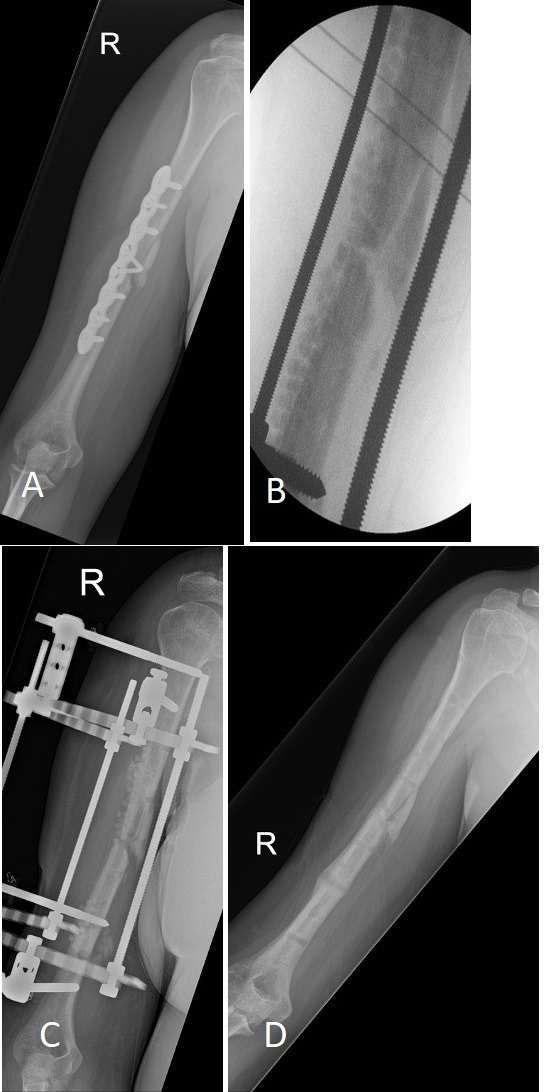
a) 39-year-old female type A host with infected nonunion of humerus fracture after open reduction and internal fixation. b) Intraoperative radiograph after plate removal, local antibiotics, and stabilization with frame. No wide resection was done. c) Interim radiograph showing good alignment and partial resorption of local antibiotics. d) Post removal of frame showing good union, no clinical signs and symptoms of recurrence of infection at 18 months after surgery.
